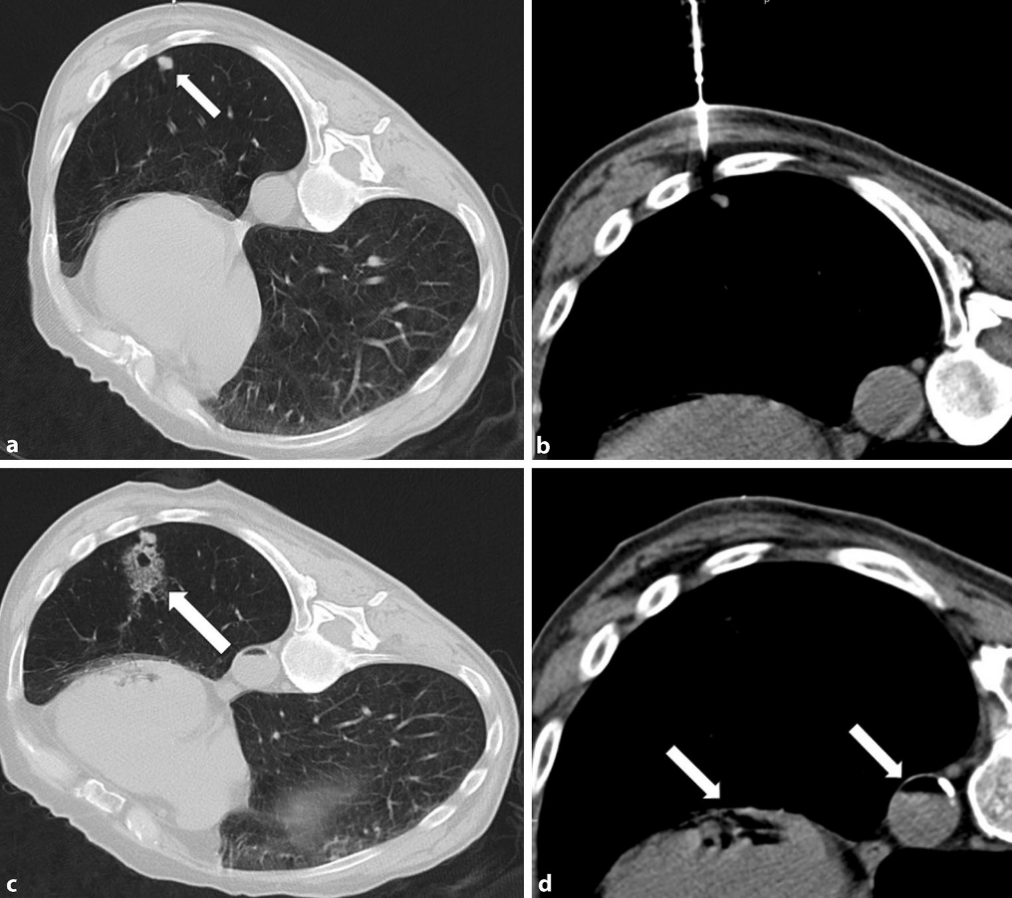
Localization of the lesion in the left lower lobe (a) and CT imaging during the intervention, b with the patient placed in prone position. The control CT scan immediately after the biopsy shows parenchymal hemorrhage (c) and an air level in the thoracic aorta and the left ventricle (c,d)
